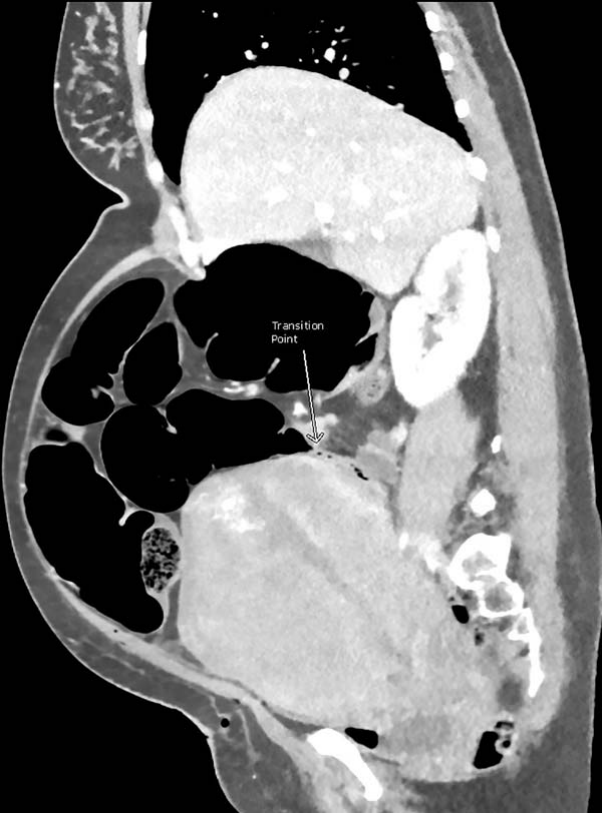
CT abdomen and pelvis with venous phase contrast, sagittal view. Abrupt collapse of the gas-filled sigmoid colon is shown posterior to the enlarged uterus. Subcutaneous gas in the anterior abdominal wall is from recent surgery.
